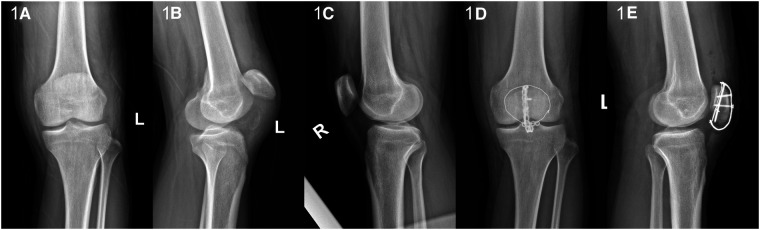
(A,B) The anterior and lateral x-ray film of knee joint of affected limb; (C) The x-ray film of healthy knee joint before operation; (D,E) the postoperative anterolateral x-ray film of knee joint.
